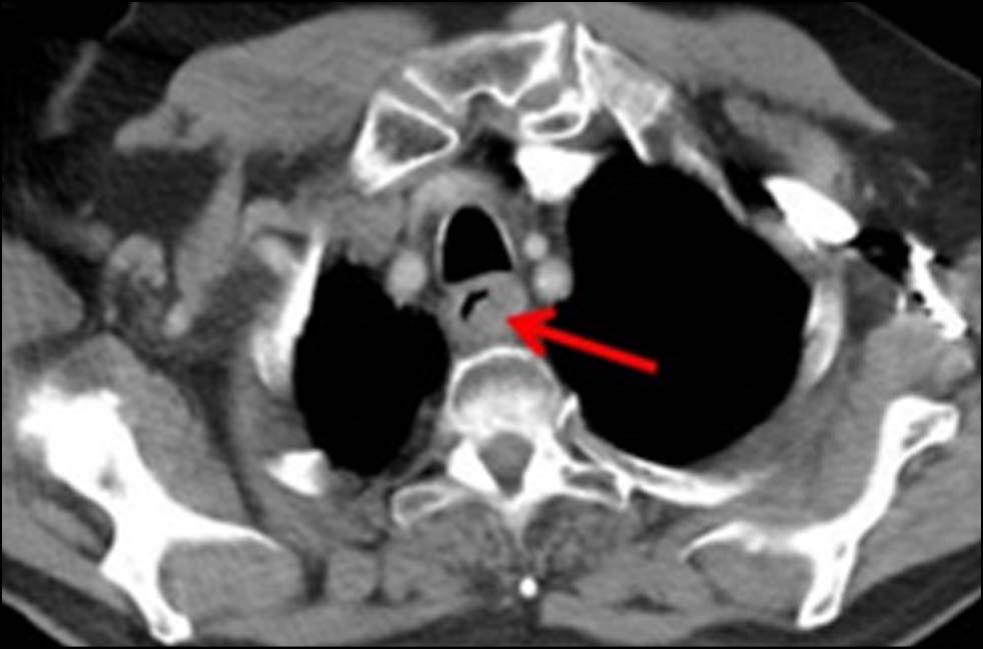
Chest CT showing soft-tissue thickening (red arrow) in the proximal third of the esophagus compatible with recurrent tumor. CT, computed tomography.
